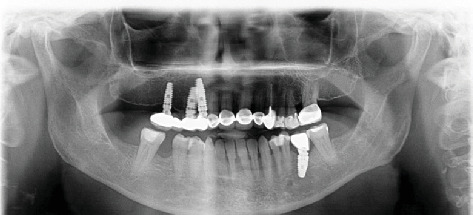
Panoramic radiograph at the 4-year follow-up.
